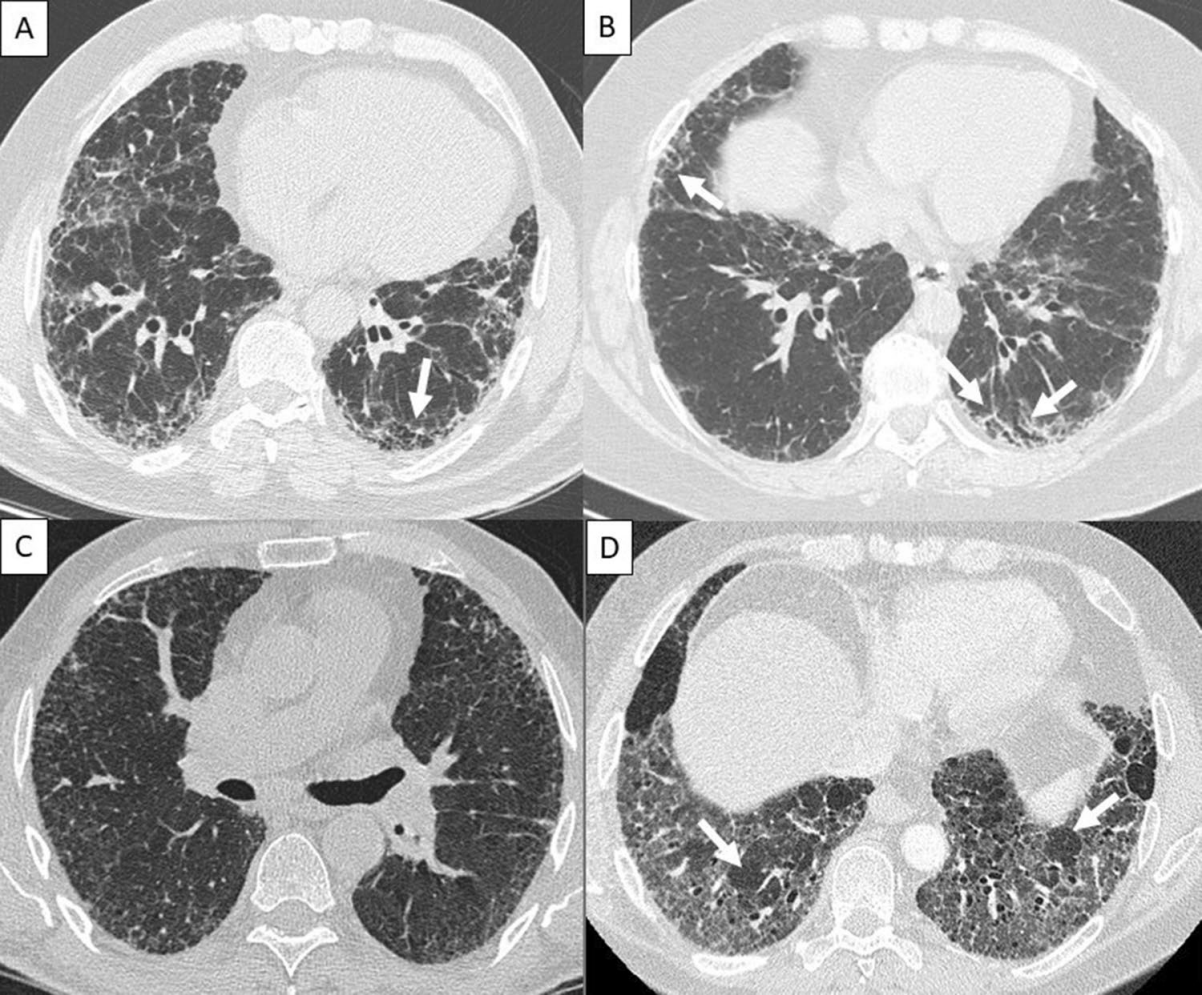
High-resolution computed tomography images of radiological patterns. (A) 67-year-old man with idiopathic pulmonary fibrosis (IPF) and usual interstitial pneumonia (UIP) pathological diagnosis. UIP radiological pattern with subpleural peripheral reticulation, traction bronchiectasis and honeycombing (arrows). (B) 66-year-old woman with IPF and UIP pathological diagnosis. Probable UIP radiological pattern with subpleural peripheral reticulation and traction bronchiectasis (arrows), without honeycombing. (C) 75-year-old man with IPF and UIP pathological diagnosis. An indeterminate pattern was seen consisting of predominating peripheral reticulation which had a diffuse distribution, shown at this section at the middle lung zone. (D) 48-year-old man with IPF and UIP pathological diagnosis. Non-UIP radiological pattern with ground-glass opacities and mosaic attenuation within reticulation areas.
